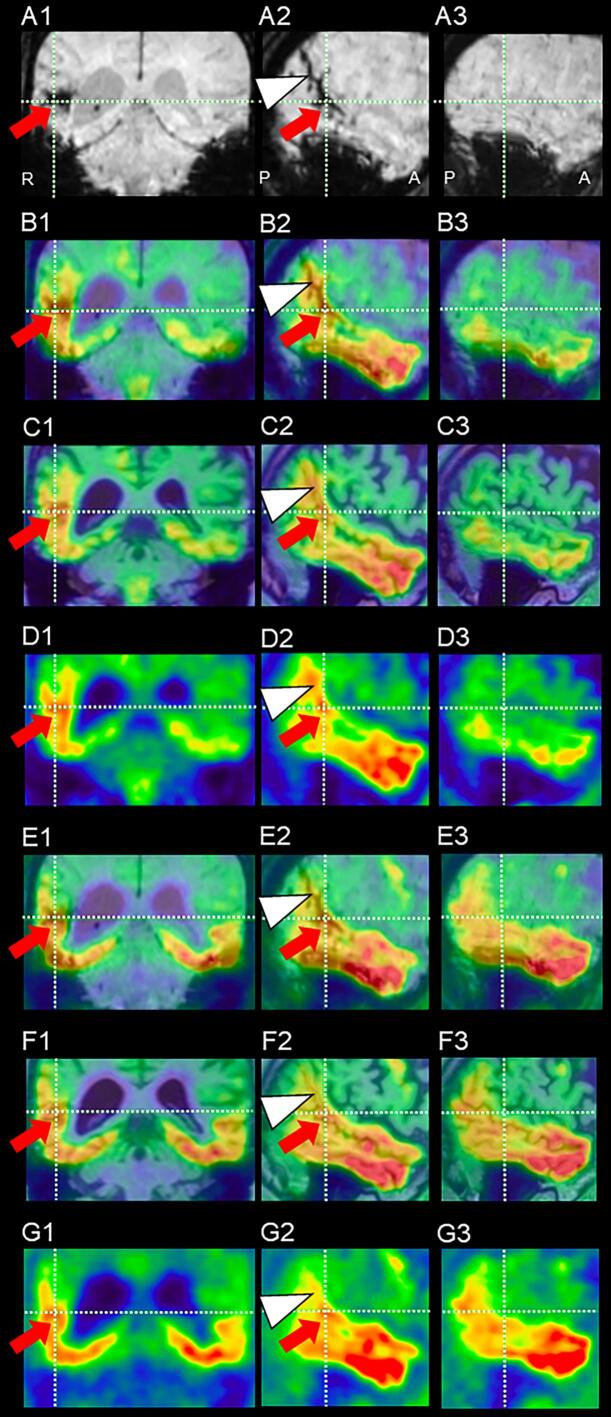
^18^F-THK5351 and ^18^F-MK-6240 positron emission tomography (PET). A. MRI SWI (X + 2/04). B–D. F-THK5351-PET (X + 2/11). E–G ^18^F-MK-6240-PET (X + 9/10). A1–G1: coronal view. A2–G2, A3–G3: sagittal view.
